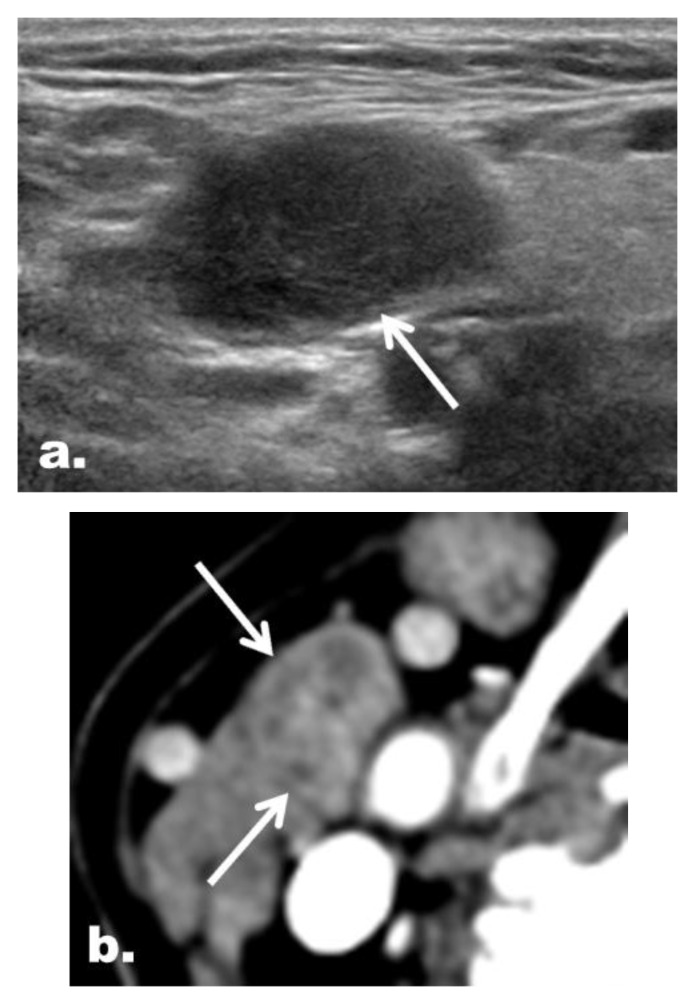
Representative case of a discordant suspicious LN with the final diagnosis of a benign LN in a 61-year-old woman with PTC. (a) On US, an ovoid LN (arrow) at the right neck level II had neither echogenic hilum nor suspicious feature and therefore was classified as an indeterminate LN. (b) On CT, LN (arrows) was interpreted to have a heterogeneous enhancement and was classified as a suspicious LN.
